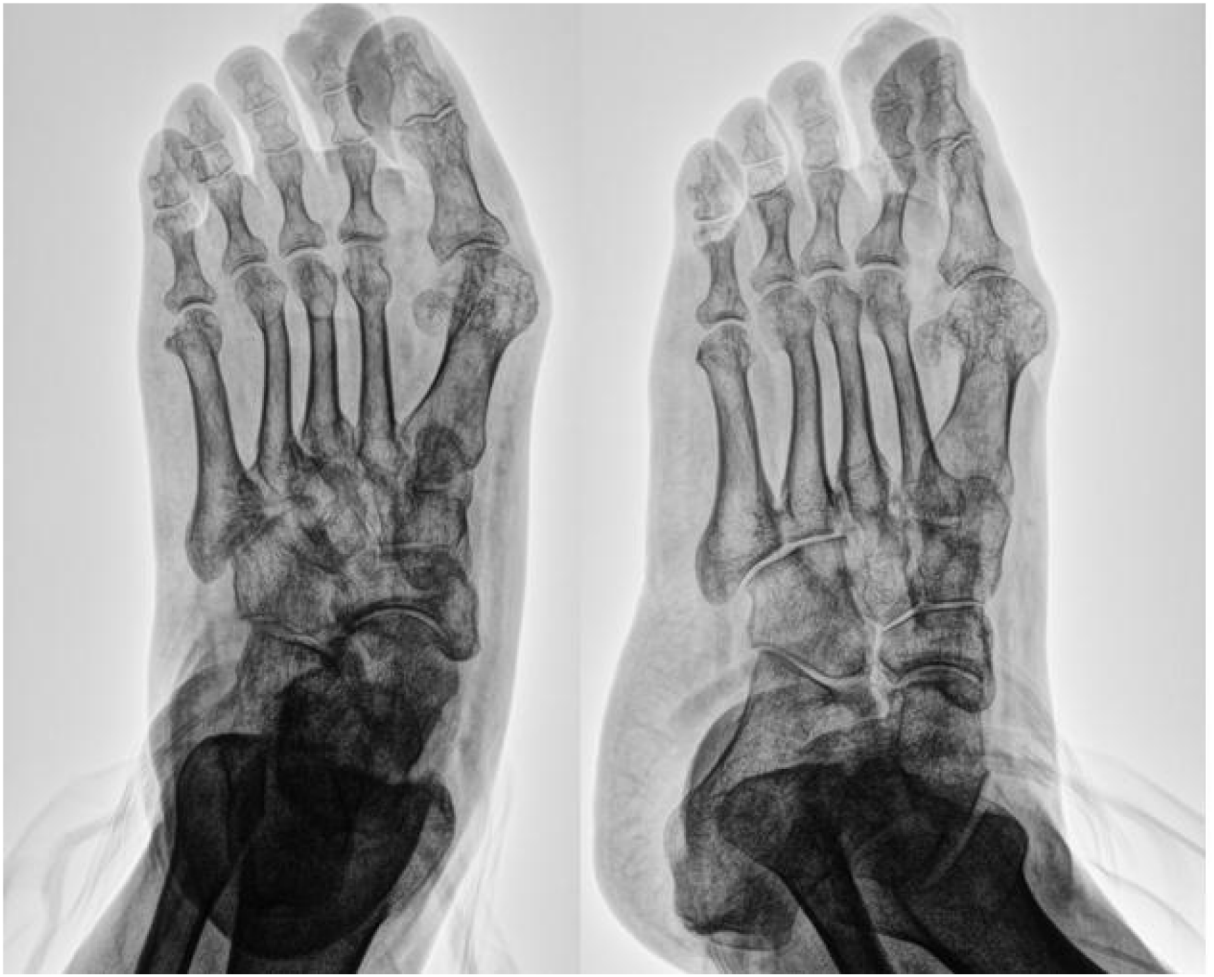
Radiograph of the left second toe showing soft tissue swelling.
